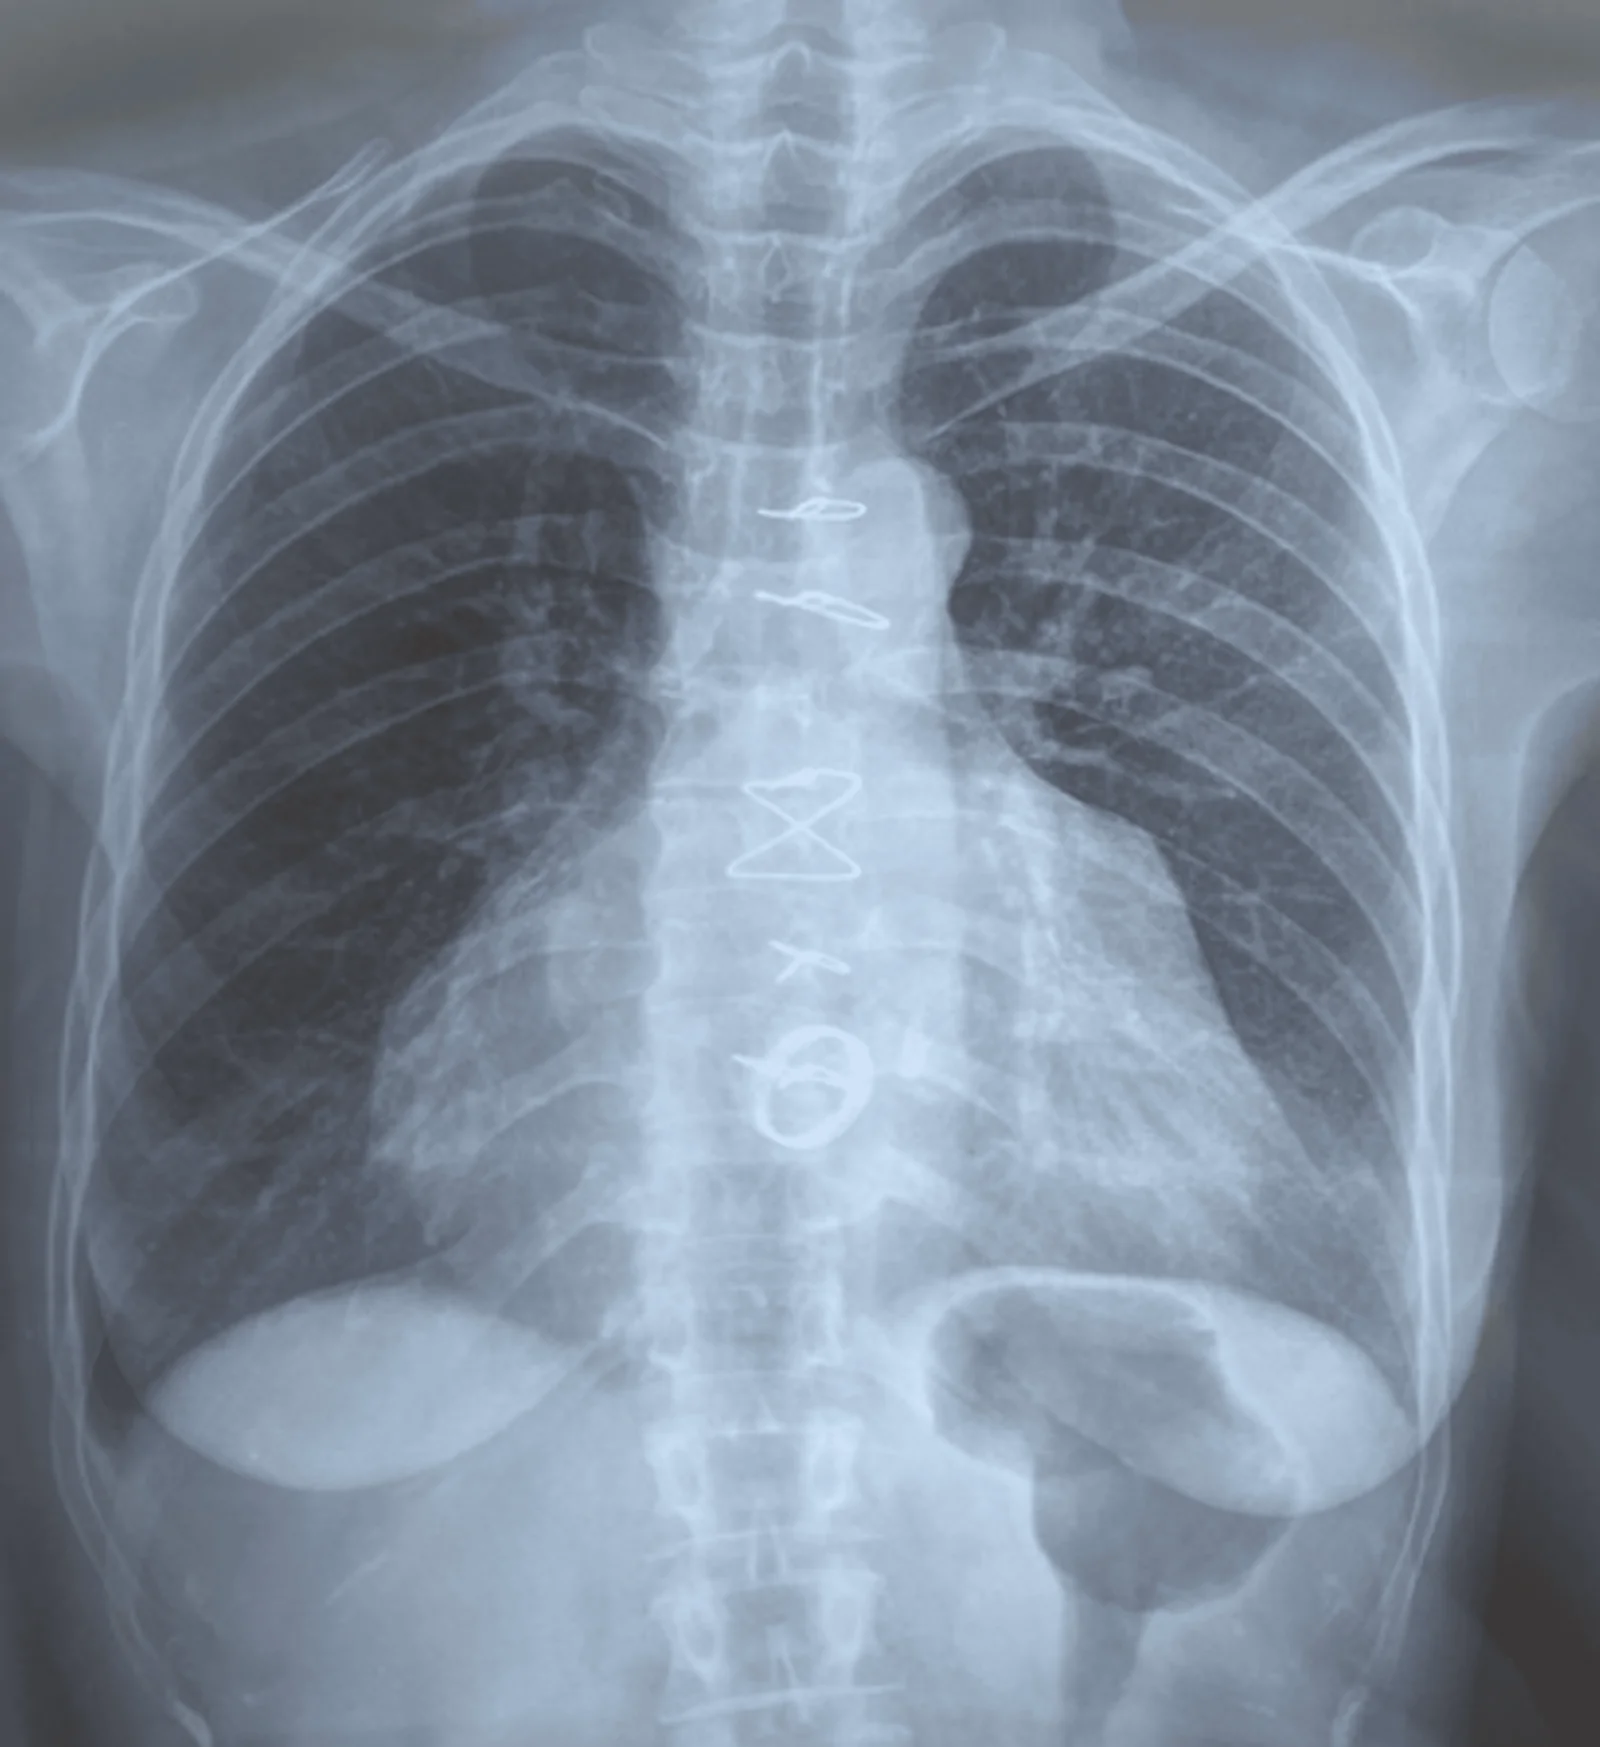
Standard chest X-ray showing cardiomegaly predominantly due to left ventricular and left atrial enlargement, with visualization of the mechanical tricuspid prosthesis and median sternotomy wires from previous cardiac surgeries.
